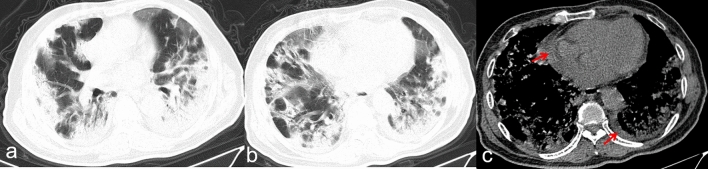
Transverse CT scans in a man infected with SARS-CoV-2 and with type 2 diabetes for 20 years. Scan obtained on 19th day from onset of symptoms shows diffuse, heterogeneous consolidation that affected bilateral lung parenchyma (a,b). The mediastinal window (c) shows a small pleural effusion at the right (arrow) and a small pericardial effusion (arrow). The patient died 4 days after this scan due to respiratory failure.
